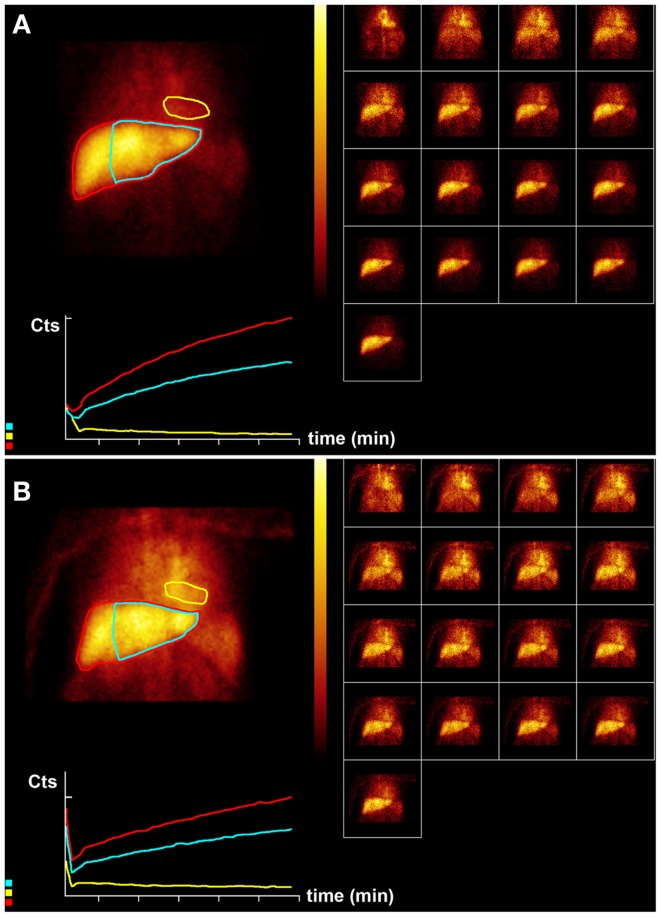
Hepatobiliary scintigraphy before (A) and 6 weeks after (B) a second treatment (6-month interval) with 1.5 GBq ^90^ Y labeled SIR-Spheres in a 65-year-old male patient with multifocal predominantly right-sided recurrent hepatocellular carcinoma (Figure 1). Displayed are summed dynamic frames (2 × 10 s/frame), a summed image (20 × 10 s) with regions of interest drawn around the entire liver (red), left liver segments 2–4 (blue), and cardiac blood pool (yellow), and corresponding time–activity curves. The total liver function (body surface area corrected mebrofenin uptake rate) was reduced after treatment from 4.8 to 2.2%/min (red curves). This time, function of left liver lobes 2–4 decreased proportionally from 3.2 to 1.6%/min (blue). The patient was diagnosed with radiation embolization induced liver disease.
